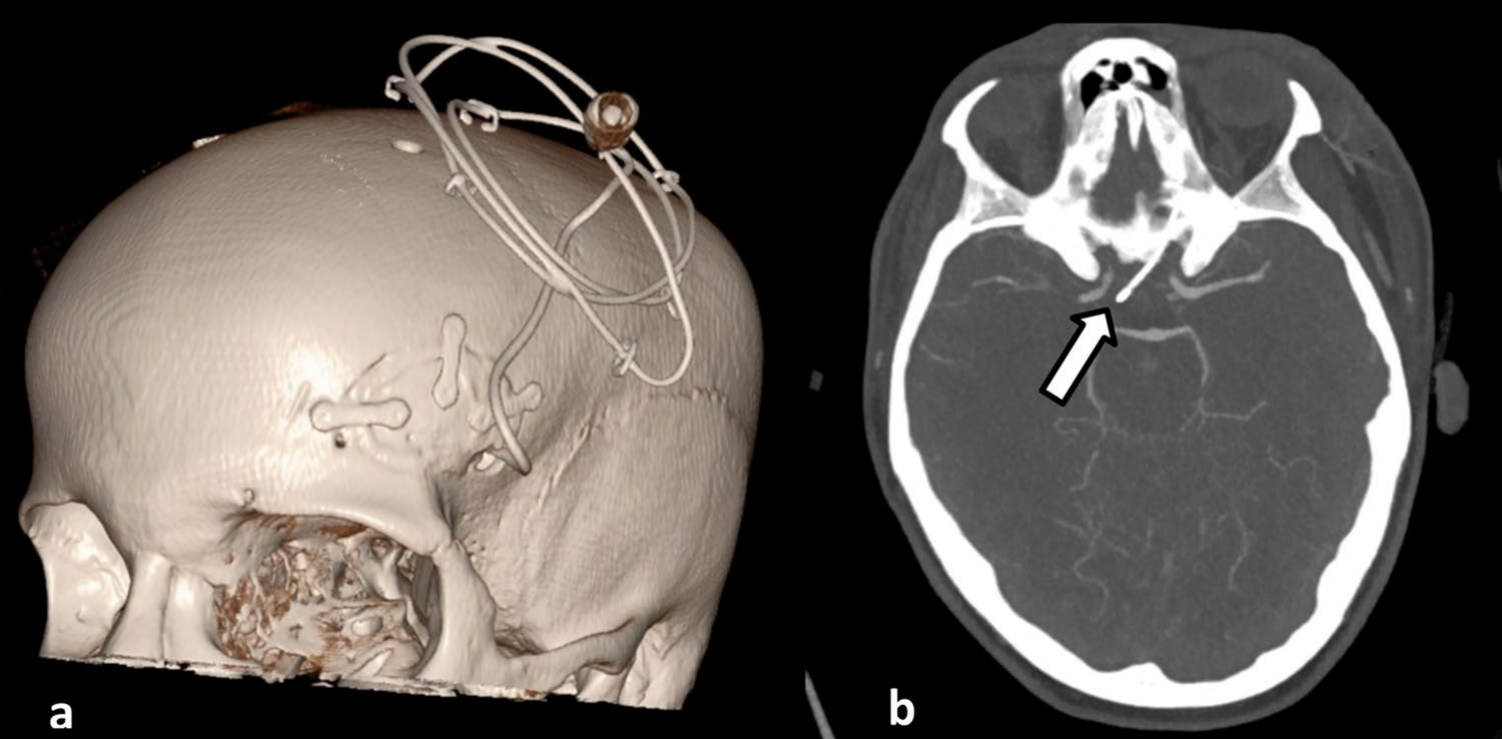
a: 3D reconstructed CT of a patient with intracisternal nimodipine catheter, which was inserted through a lateral supraorbital mini-craniotomy. b: axial CT showing the intracranial position of the nimodipine catheter in the optico-carotid cistern (white arrow)
